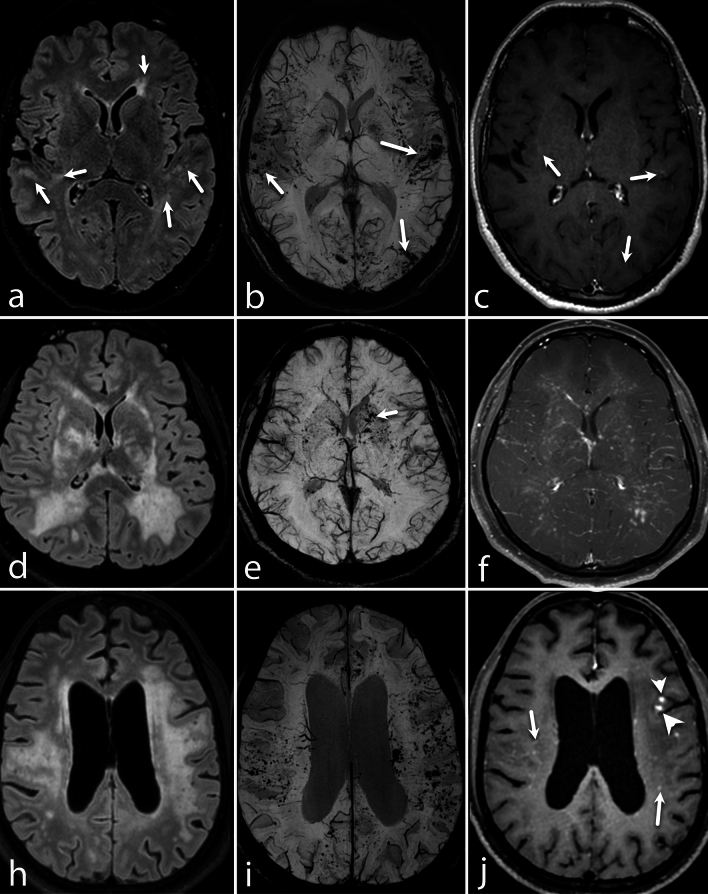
Different patterns of FLAIR hyperintensities, SWI hemorrhages, and enhancements in PCNSV. (a–c) A 28-year-old man presented with headaches for the past 3.5 years. The axial FLAIR (a) image shows multi-focal subtle areas of FLAIR hyperintensities in subcortical and periventricular white matter (arrows) of bilateral cerebral hemispheres. The axial SWI image (b) shows multiple microhemorrhages (arrows) in bilateral cerebral hemispheres. Axial post-gadolinium T1-WI (c) shows punctate foci of enhancement (arrows) in bilateral cerebral hemispheres. (d–f) A 23-year-old man presented with acute onset vision loss in the left eye. The axial FLAIR image (d) shows confluent symmetrical pattern of white matter hyperintensity in the periventricular white matter of the bilateral fronto-parietal lobe, basal ganglia, internal capsule, and external capsule. The axial SWI (e) shows multiple foci of microhemorrhages (arrow) predominantly in bilateral basal ganglia. Post-contrast image (f) shows a diffuse perivascular and nodular enhancement pattern in the bilateral cerebral hemisphere. (g–i) A 37-year-old man presented with behavioral changes for the past 6 months. The axial FLAIR (g) image shows large confluent and discrete areas of periventricular and subcortical white matter hyperintensity in bilateral frontoparietal lobes. The axial SWI (b) shows diffuse punctate foci of microhemorrhages in the bilateral cerebral hemisphere. On the post-contrast image (c), a few nodular (arrowheads) and punctate foci (arrows) of enhancement are seen.
